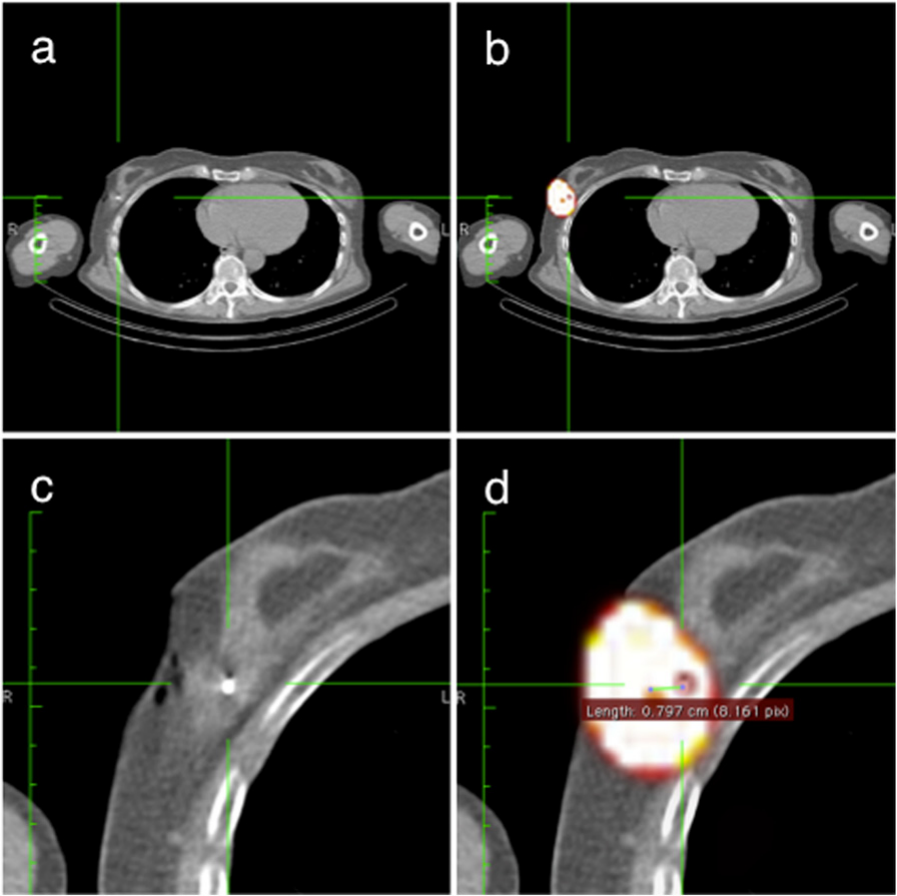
Axial SPECT/CT images. (a) The low-dose CT. (b) The SPECT and CT images fused. (c) Close-up of the low-dose CT. (d) The SPECT and CT images fused with the measurement of distance from the centre of activity of the ^99m^Tc-nanocolloid to the ^125^I seed; the measured distance is 8 mm. The green lines indicate the same position in the different images.
